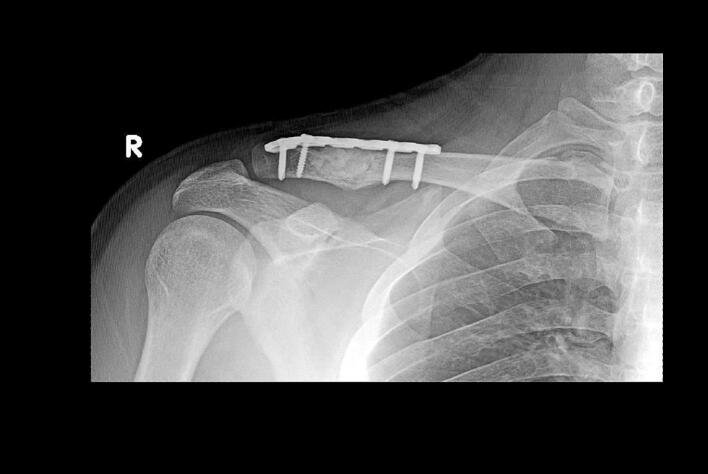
Post-operative radiographs showed good alignment with minimal but increased callous formation. No recurrent mass was seen.
